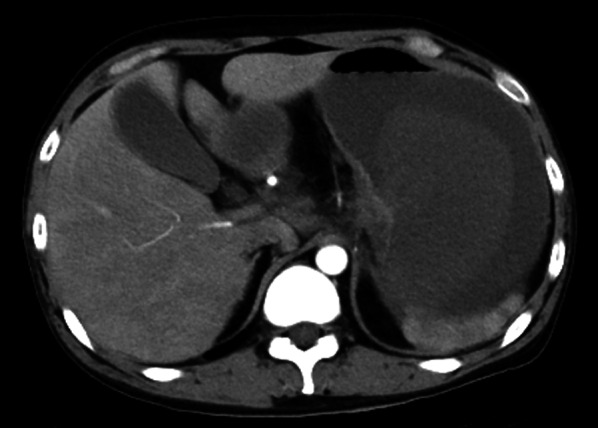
Dynamic computed tomography (CT) shows a massive, contrast-enhanced fluid collection in the stomach
